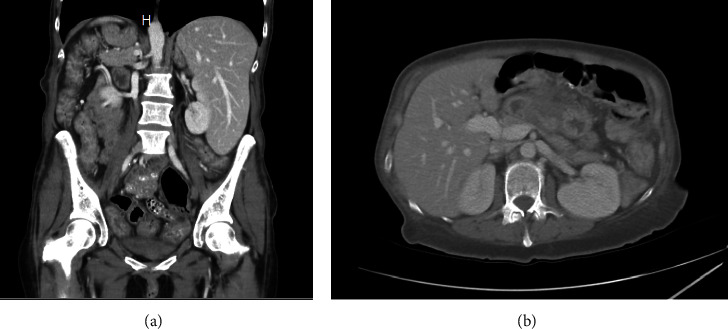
(a, b) CT scan showing mild colitis in the transverse and descending colon.
